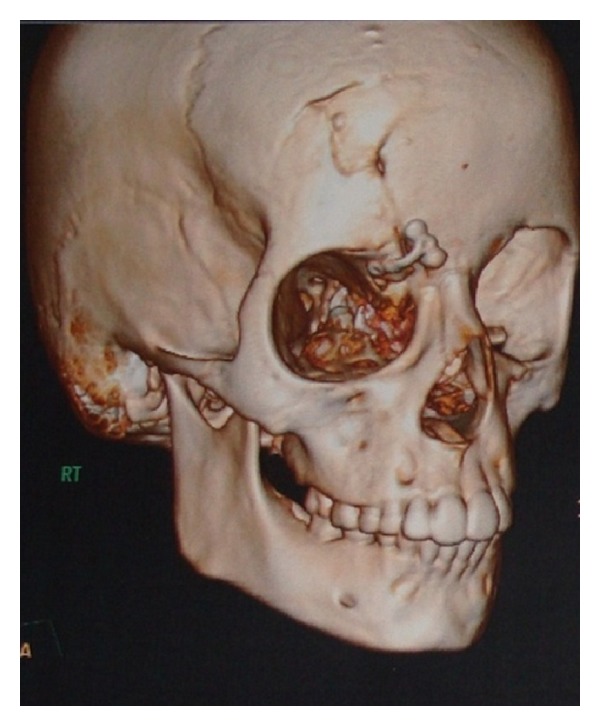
Postoperative 3D CT scan revealing titanium plate and screws intact at the fracture site.
